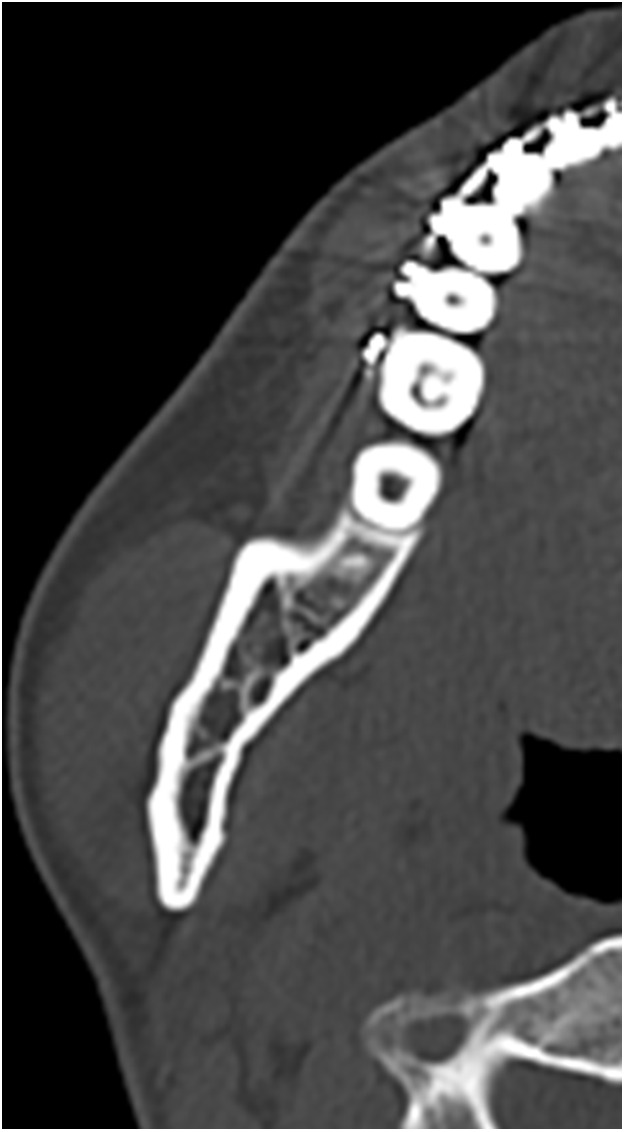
Axial computed tomography images showing no contact between the mandibular canal and the lateral cortical bone.
